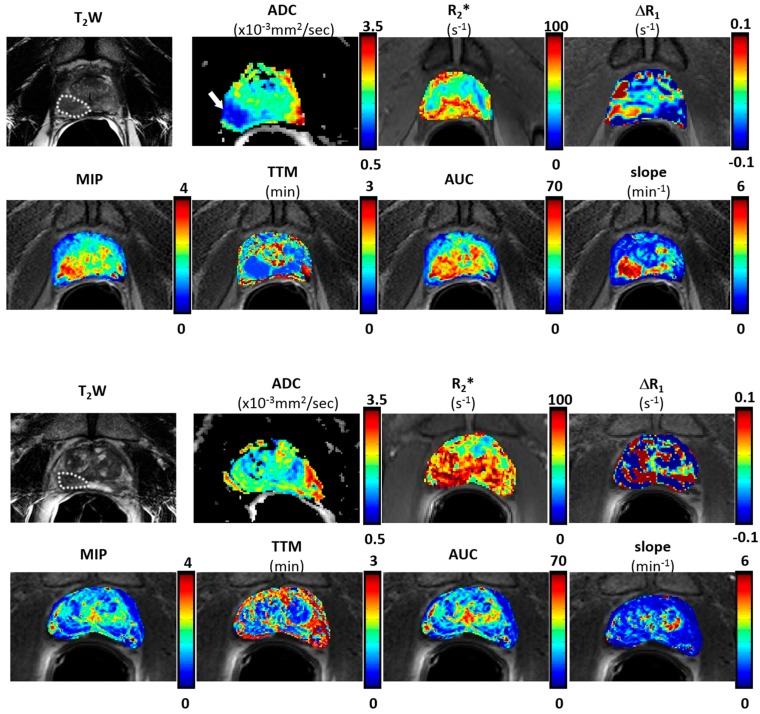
Multi-parametric MRI maps of two patients with Gleason score 8 (top panel) and 6 (bottom panel) tumors respectively. Parametric maps of prostate observed with endorectal coil at 3 T. For the patient with high Gleason score, the tumor region (white dotted lines) showed relatively higher values of R_2_* (air), maximum-intensity-projection (MIP), area-under-the-curve (AUC) and slope maps, while lower in apparent diffusion coefficient (ADC) and time-to-maximum (TTM) maps. No obvious difference was observed for the patient with a low Gleason score.
